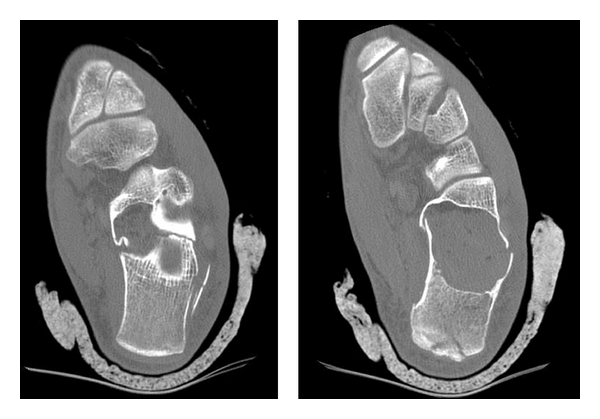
CT scan views on the transverse plane showing an undisplaced pathologic fracture over a simple calcaneal cyst.
